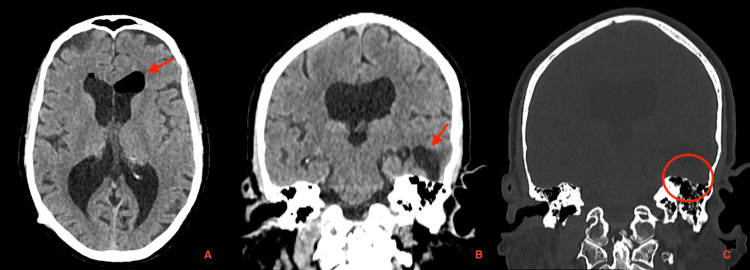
Head CT scan demonstrating spontaneous intraventricular pneumocephalus (red arrow, image A) and a region of left temporal encephalomalacia with a cystic, CSF-filled appearance (red arrow, image B). A small air bubble is observed adjacent to the mastoid tegmen, suggesting a possible mastoid bone defect (red circle, image C). CT: computed tomography; CSF: cerebrospinal fluid
